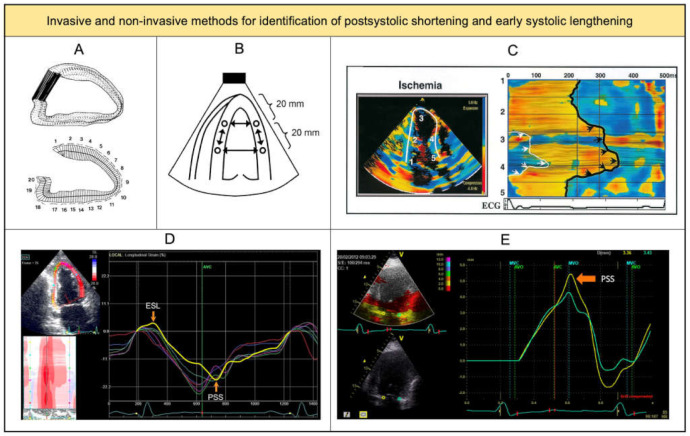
Invasive and non-invasive methods for assessing deformation patterns. Legend: (A) Ventriculogram depicting the centerline method for assessing wall motion. (B) Circles in the myocardial walls indicate embedded ultrasonic crystals to determine segmental length during the cardiac cycle. (C) Strain rate map during ischemia, where black arrows indicate postsystolic shortening and white arrows indicate delayed systolic shortening. (D) Longitudinal speckle tracking profile showing early systolic lengthening and postsystolic shortening. (E) Tissue Doppler imaging of longitudinal displacement of the mitral valve annulus showing postsystolic shortening. (A–C) are reprinted from Hosokawa et al. [14], Amundsen et al. [15] and Pislaru et al. [16] with permission the American College of Cardiology (Elsevier). (D) is reprinted from Asanuma et al. [6] with permission from the British Medical Journal Publishing Group. (E) is reprinted from Brainin et al. [17] with permission from Springer Nature.
